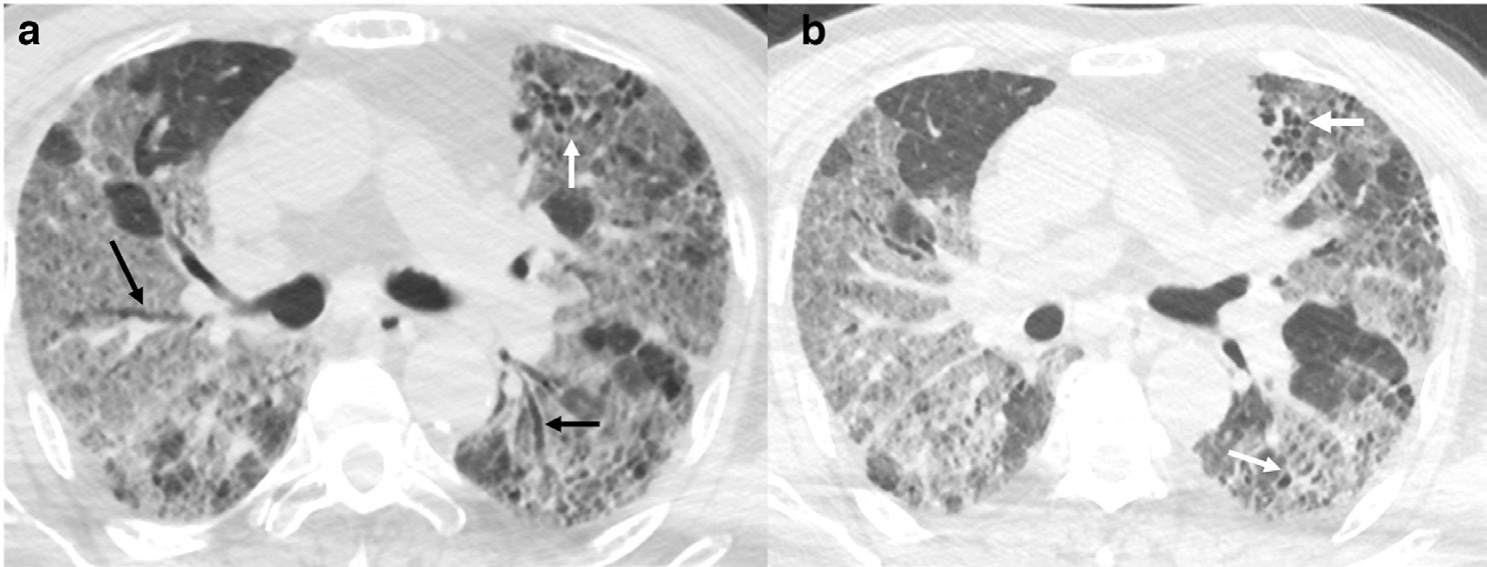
76-year-old male with COVID-19 pneumonia with CT done on the day of diagnosis. Axial images (A, B) show diffuse bilateral GGO due to acute infection. Additionally, there is bronchiectasis (black arrows) and peripheral bronchiolectasis and honey-combing (white arrows) due to underlying fibrosis.The early timeline of the infection helped to differentiate the volume loss changes from resportivephase of COVID-19 pneumonia. GGO, ground-glassopacity.
